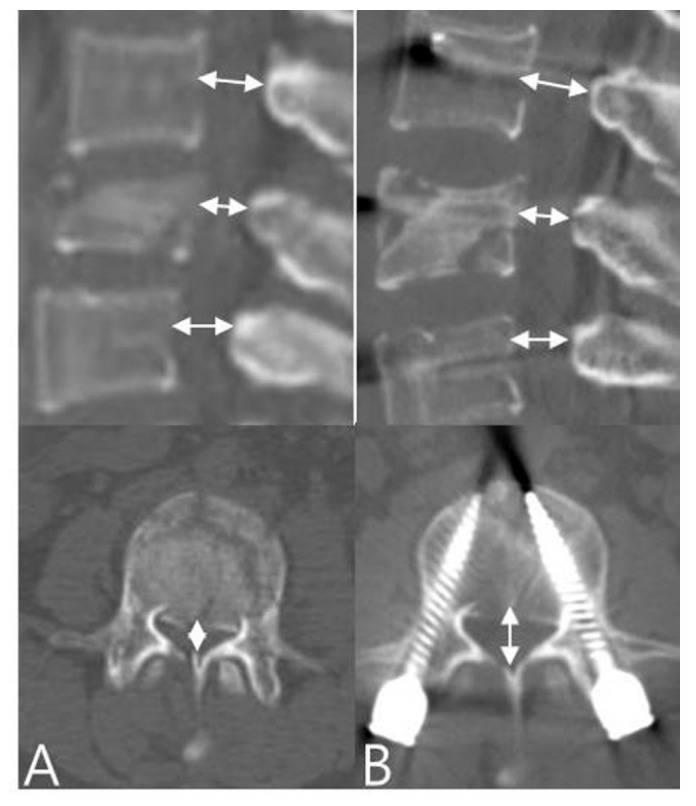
Spinal canal encroachment and clearance were calculated (A) before and (B) after sur gery on CT scans. The percentage of canal compromise was calculated with the narrowest mid-sagittal diameter of the injured level divided by the mean of the mid-sagittal diameters of the adjacent upper and lower vertebrae.
